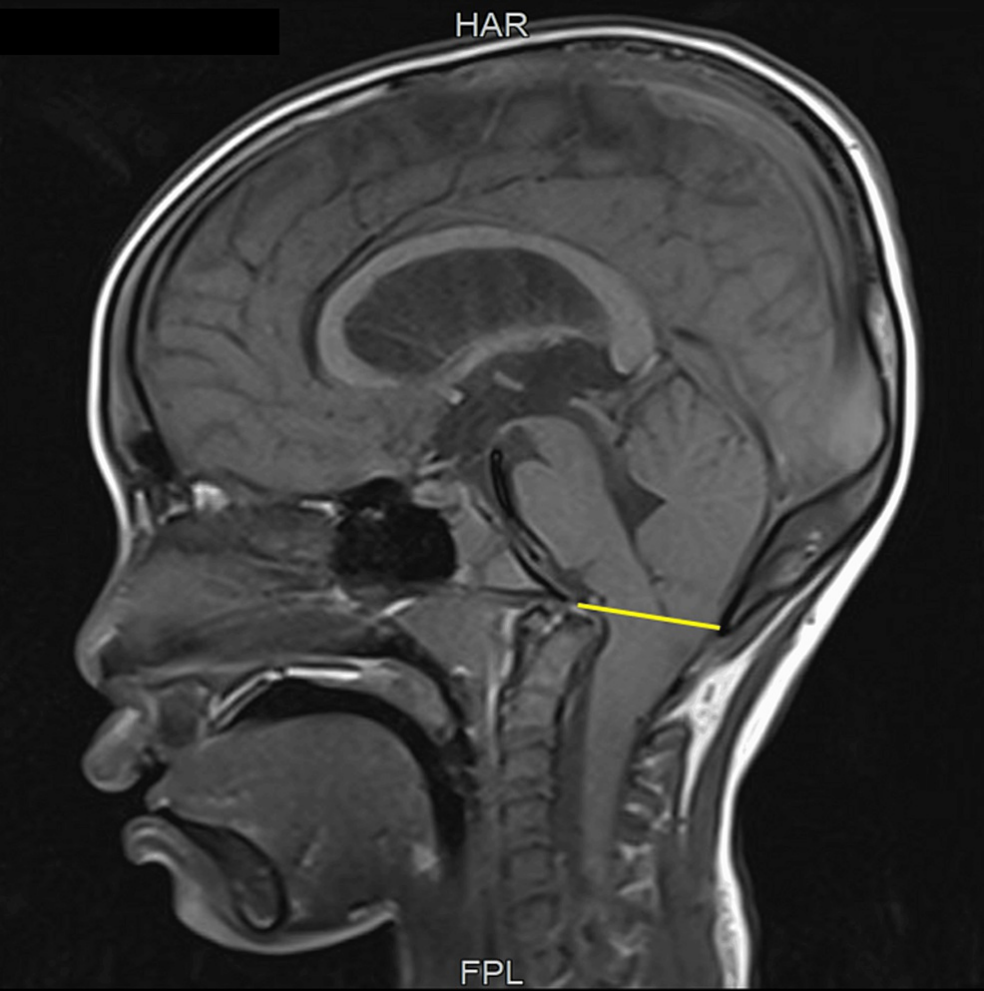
MRI showing the downward displacement of the cerebellum below the McRae line (yellow) indicating a Chiari malformation MRI: magnetic resonance imaging
